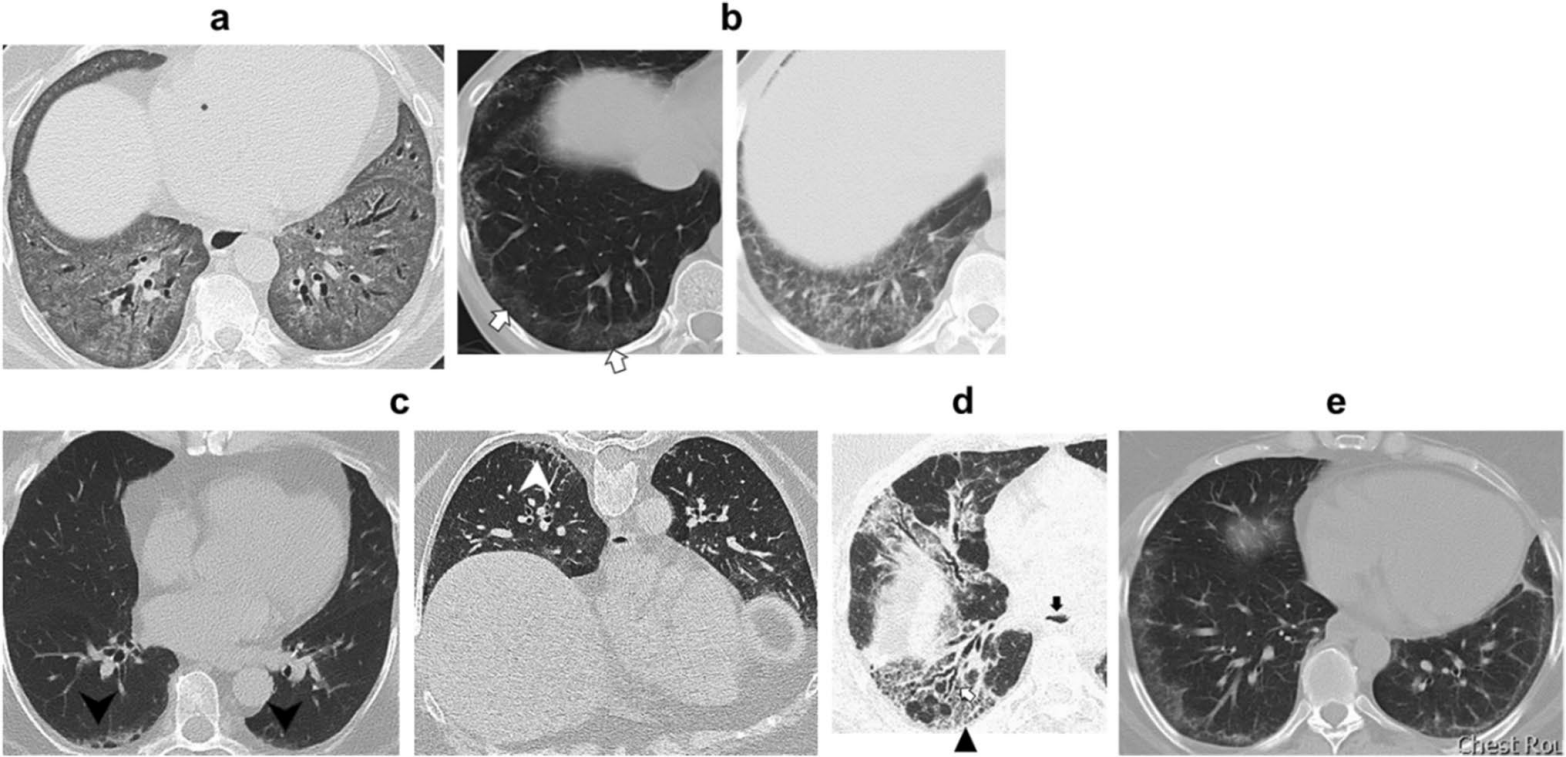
a Chest CT scan of a 52-year-old female with SSc demonstrating lower lobe–predominant diffuse ground-glass opacities suggestive of NSIP imaging pattern. b Unenhanced axial chest CT images of two patients with SSc-ILD showing lower lobe–predominant ground-glass opacity, the dominant feature of NSIP. In the image on the left, ground-glass opacities in the lower lobes are peripheral in distribution (white arrows); these are more diffuse in the image on the right. c Dependent opacities (black arrowheads) in the lung bases of a patient with SSc that persist on the right on the prone image (white arrowhead), suggesting early interstitial fibrosis. d Unenhanced axial chest CT image of a 57-year-old female with SSc-ILD showing ground-glass opacities and reticular changes in a peribronchovascular (white arrowhead) and subpleural (black arrowhead) distribution in the lower lobe. The black arrow indicates a dilated esophagus. e Chest CT scan showing subpleural sparing. CT, computed tomography; NSIP, non-specific interstitial pneumonia; SSc-ILD, systemic sclerosis-associated interstitial lung disease
